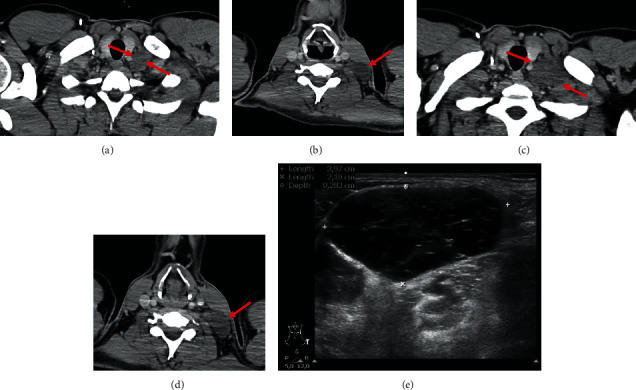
CT and ultrasound. Axial thoracic contrast-enhanced CT images at 2-month (a, b) and 5-month follow-up (c, d), showing progressive enlargement of left cervical lymph nodes with diffuse hypodensity (red arrows). (d) Ultrasound image of the same left cervical lymph node depicted on the CT images (a–d) at the microbiopsy, which showed mixed echogenicity, being primarily hypoechogenic with some echogenic septa.
